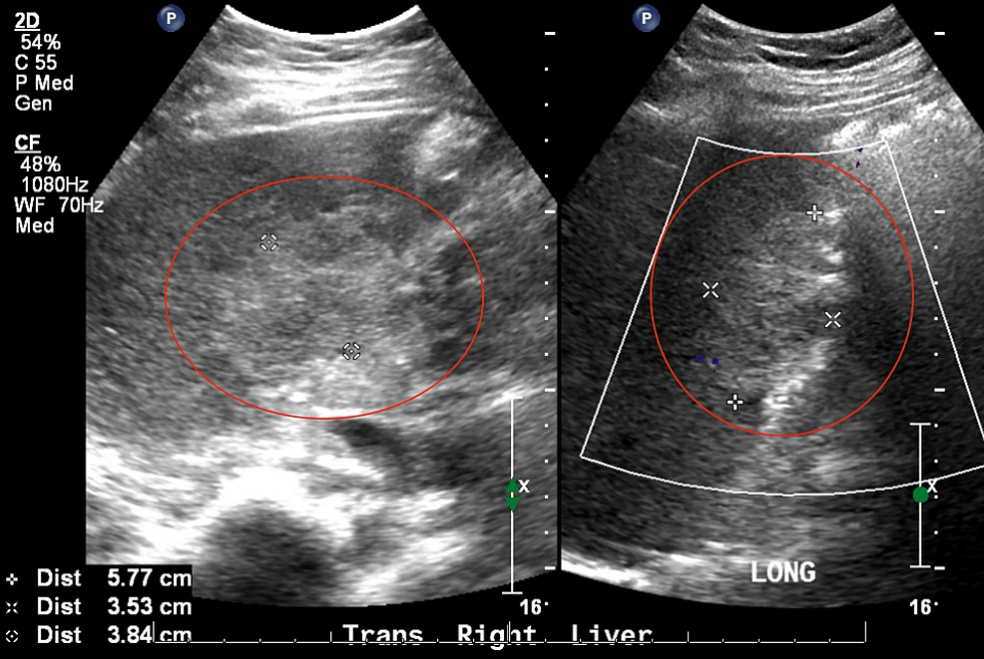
Abdominal ultrasound with Doppler showed a large right hyper-echoic hepatic lesion (red circles) and mildly increased overall hepatic parenchymal echogenicity.
